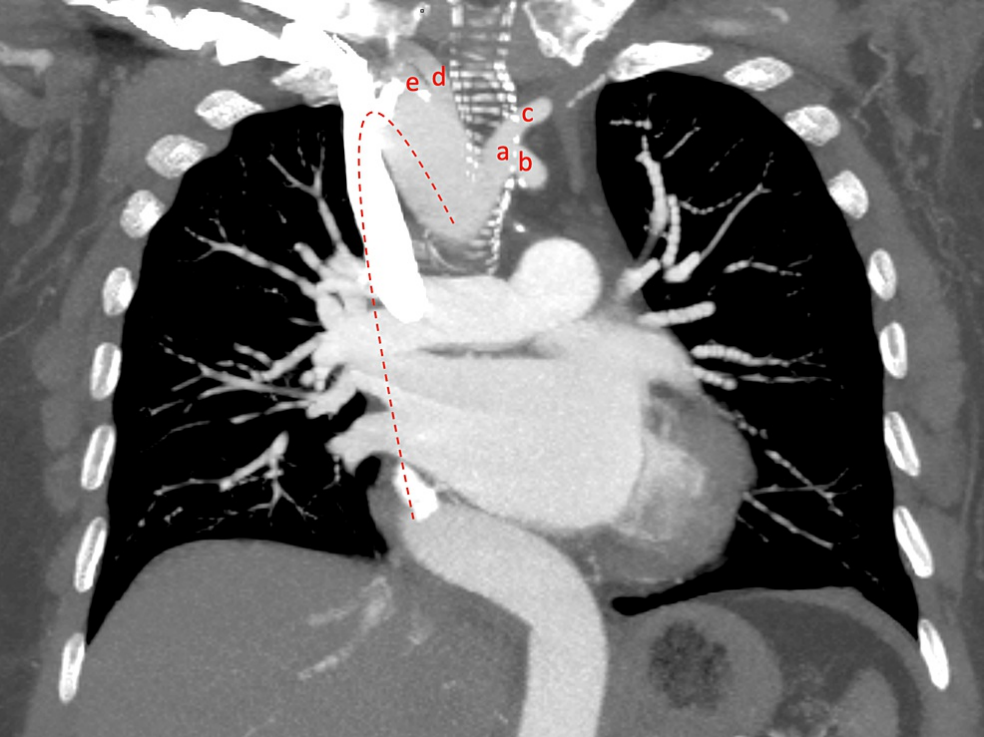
Computed tomography angiography showing the right-sided orientation of the aortic arch (red dashed line) with mirror-image branching. Aortic arch branches from proximal to distal: a: left innominate artery, b: left subclavian artery, c: left common carotid artery, d: right common carotid artery, e: right subclavian artery.
